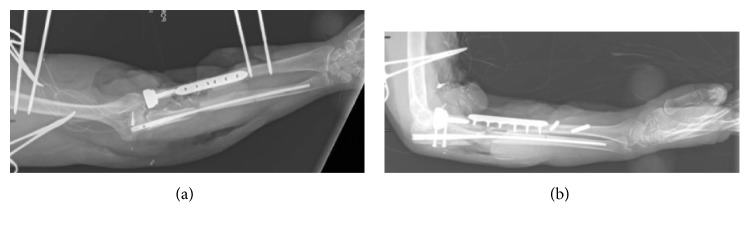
AP and lateral X-rays of the patient's forearm on POD 75. The ulnar has an IMN nail, and the radius has an open reduction internal fixation of the fibular free graft demonstrating good alignment.
